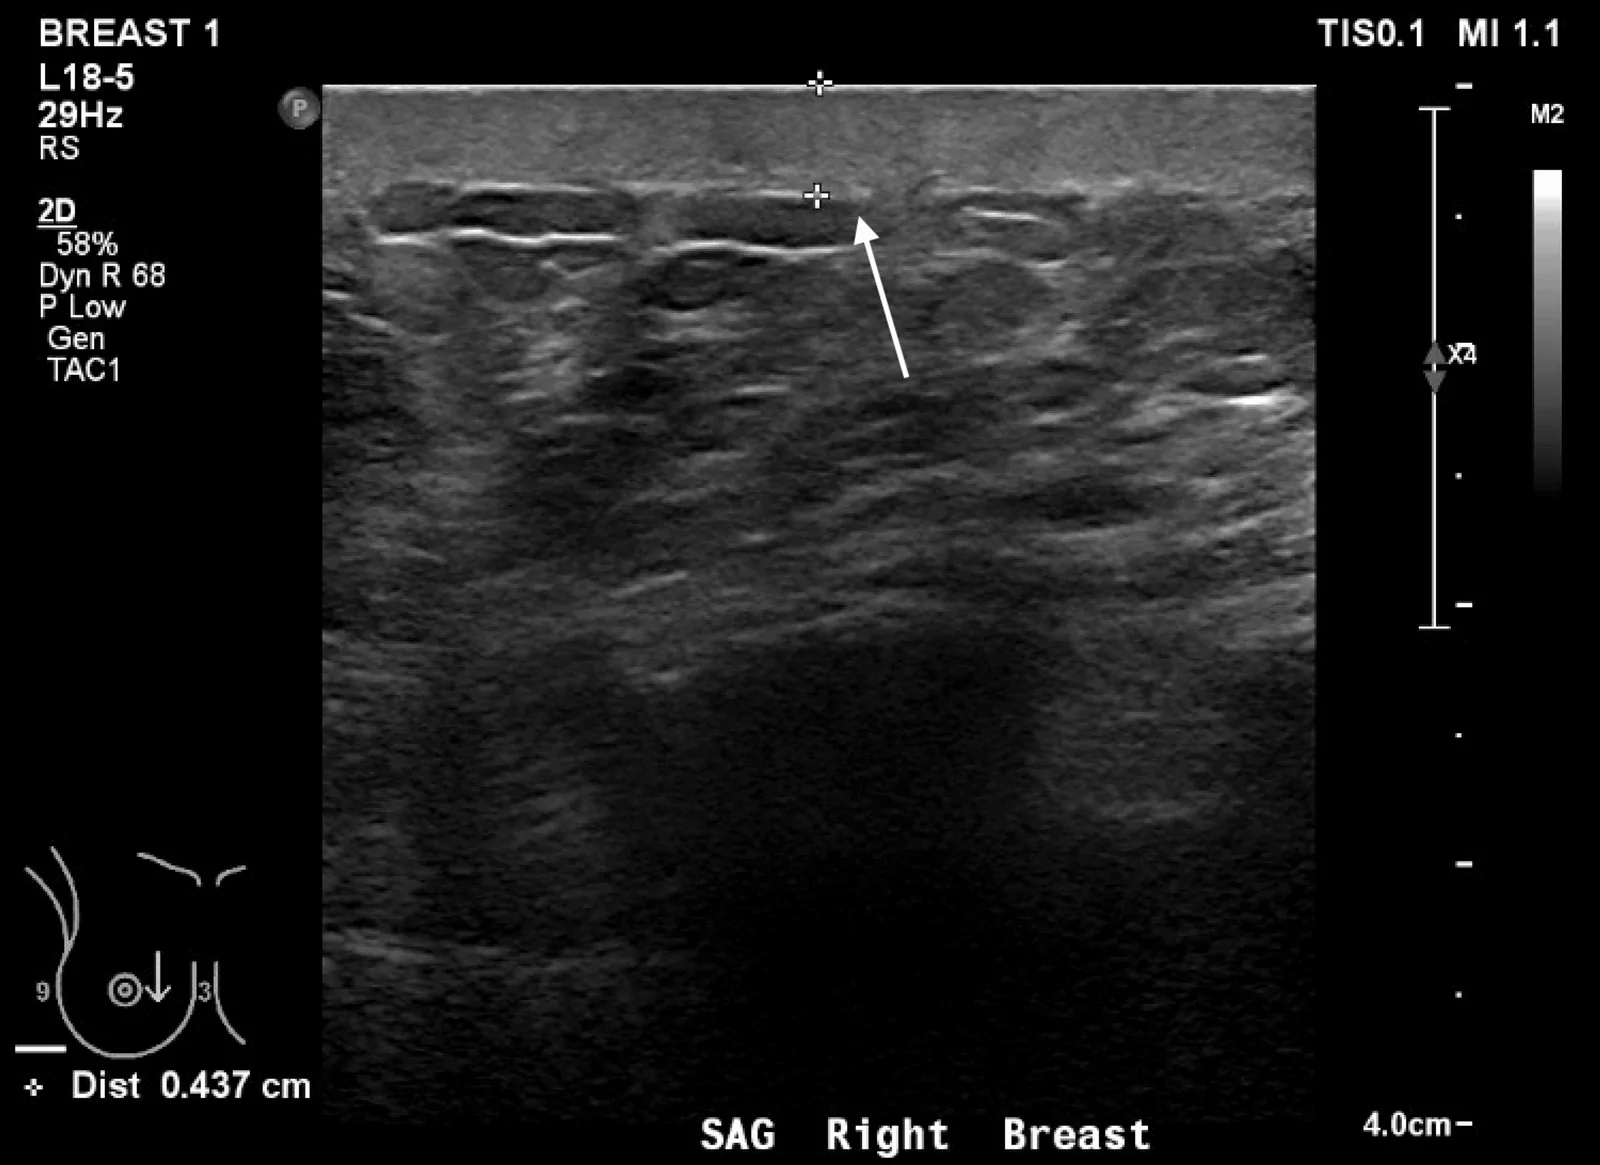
Pretreatment ultrasound Targeted ultrasound of the right breast demonstrating abnormal skin thickening, corroborating the clinical findings of diffuse skin involvement.
